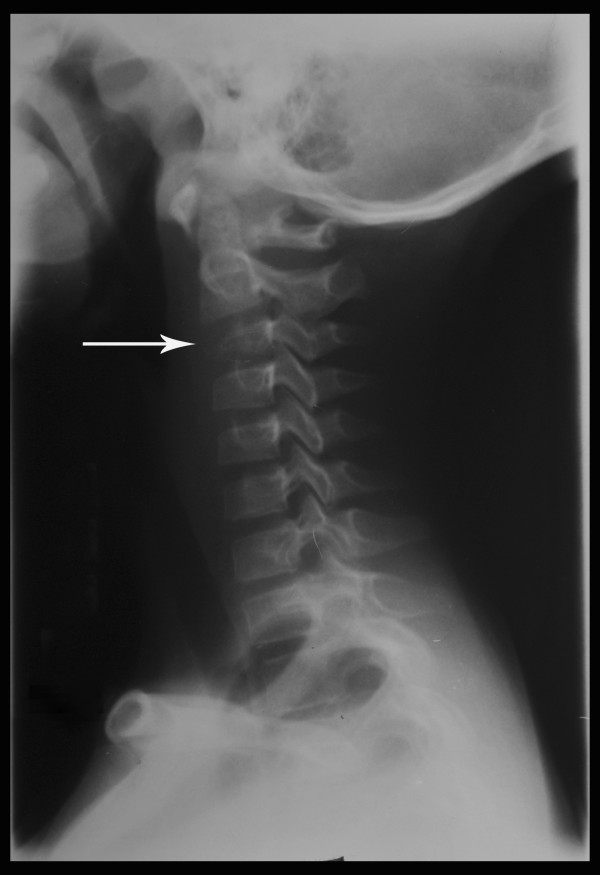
Plain radiograph showing straight spine with destruction and collapse of third cervical vertebra anteriorly (arrow) and normal intervertebral disc spaces.
